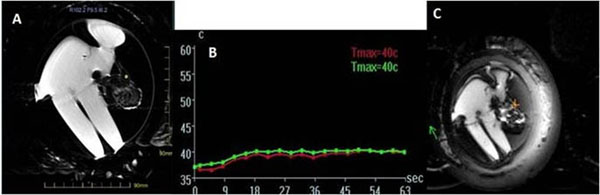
Example of Thermal Monitoring during sonication: Coronal MR image showing planned sonication location (A); temperature graph during sonication (B); the anatomical image acquired during sonication (C)
